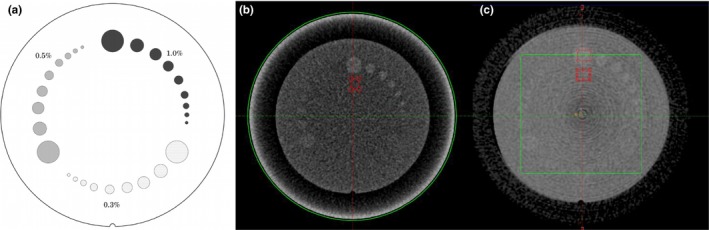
Quantitative measurement of contrast‐to‐noise ratio. (a) Catphan model 604 low‐contrast section. (b) Measurement of 15‐mm diameter circle with 1% contrast object using original CT head & neck protocol. (c) Measurement using CBCT pelvis imaging protocol on same locations. CBCT, cone‐beam computed tomography.
